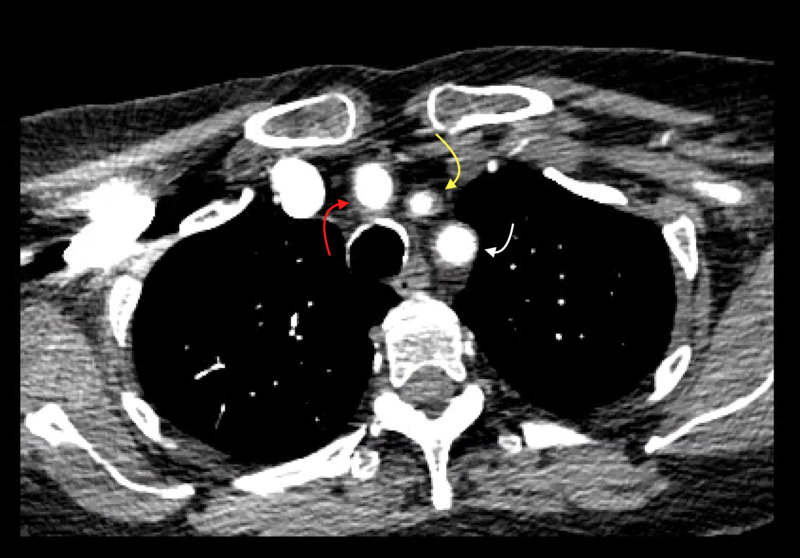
CT angiogram showed wall thickening of the brachiocephalic (red arrow), left common carotid (yellow arrow), and left subclavian (white arrow) arteries suggestive of arteritis
